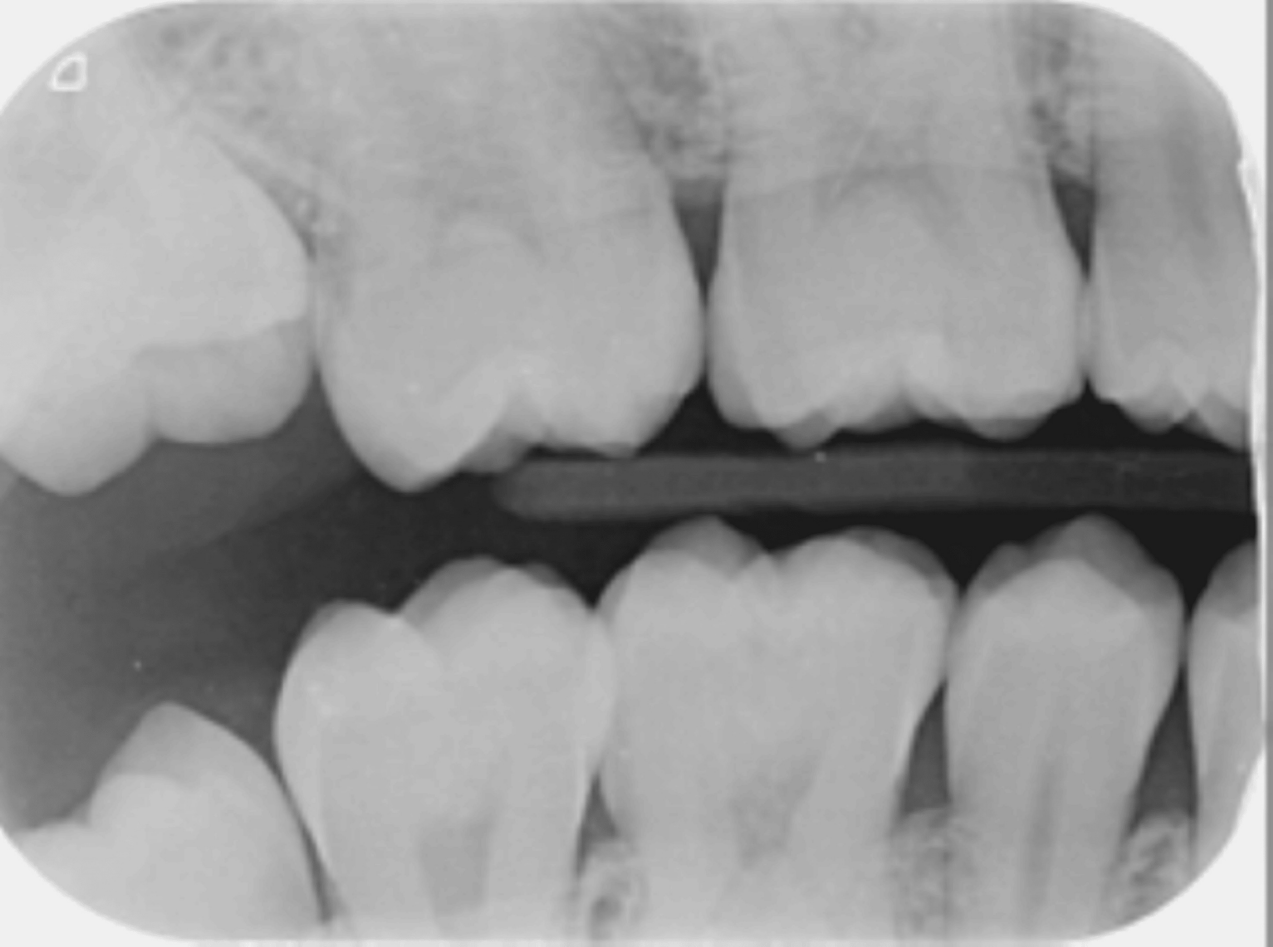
Bitewing radiograph of right quadrant.
